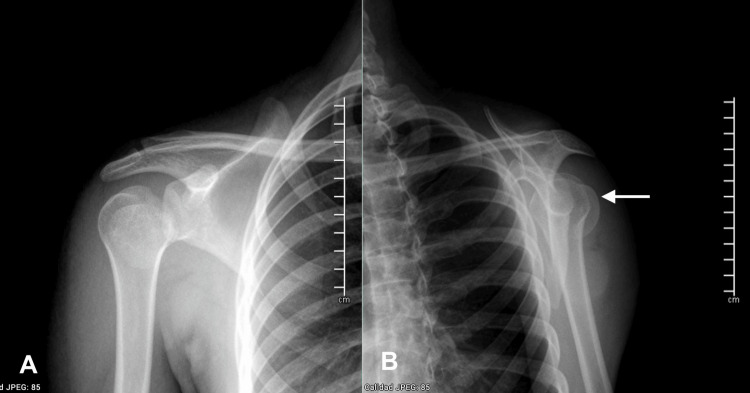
Initial anteroposterior (A) and axial (B) radiographs The white arrow indicates a bone fragment in the posterior situation.
